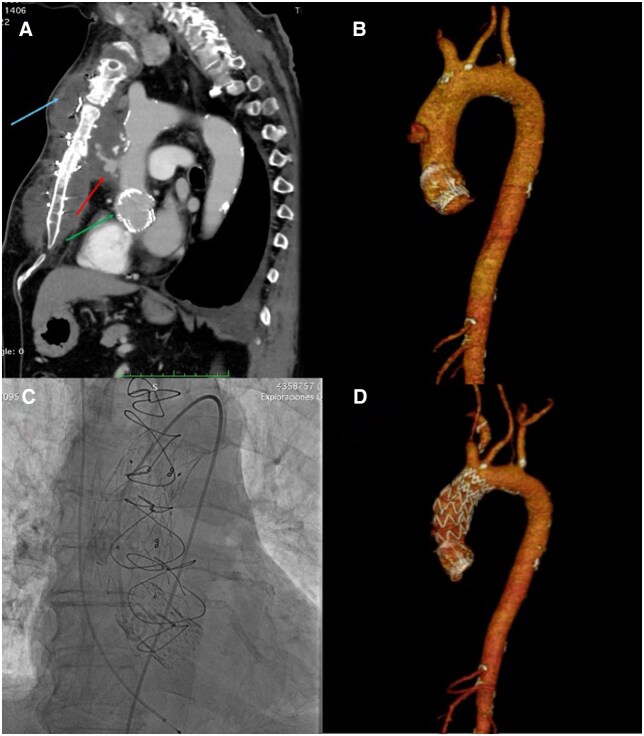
Preoperative, Intraoperative, and Postoperative Imaging of Patient no. 3. (A) CTA Showing Presternal Hematoma, Ascending Pseudoaneurysm, and Perceval Valve. (B) 3D CT Reconstruction. (C) Fluoroscopy during Transfemoral Nexus Ascending Module Deployment. (D) Postoperative CTA Confirming Exclusion without Endoleak.
